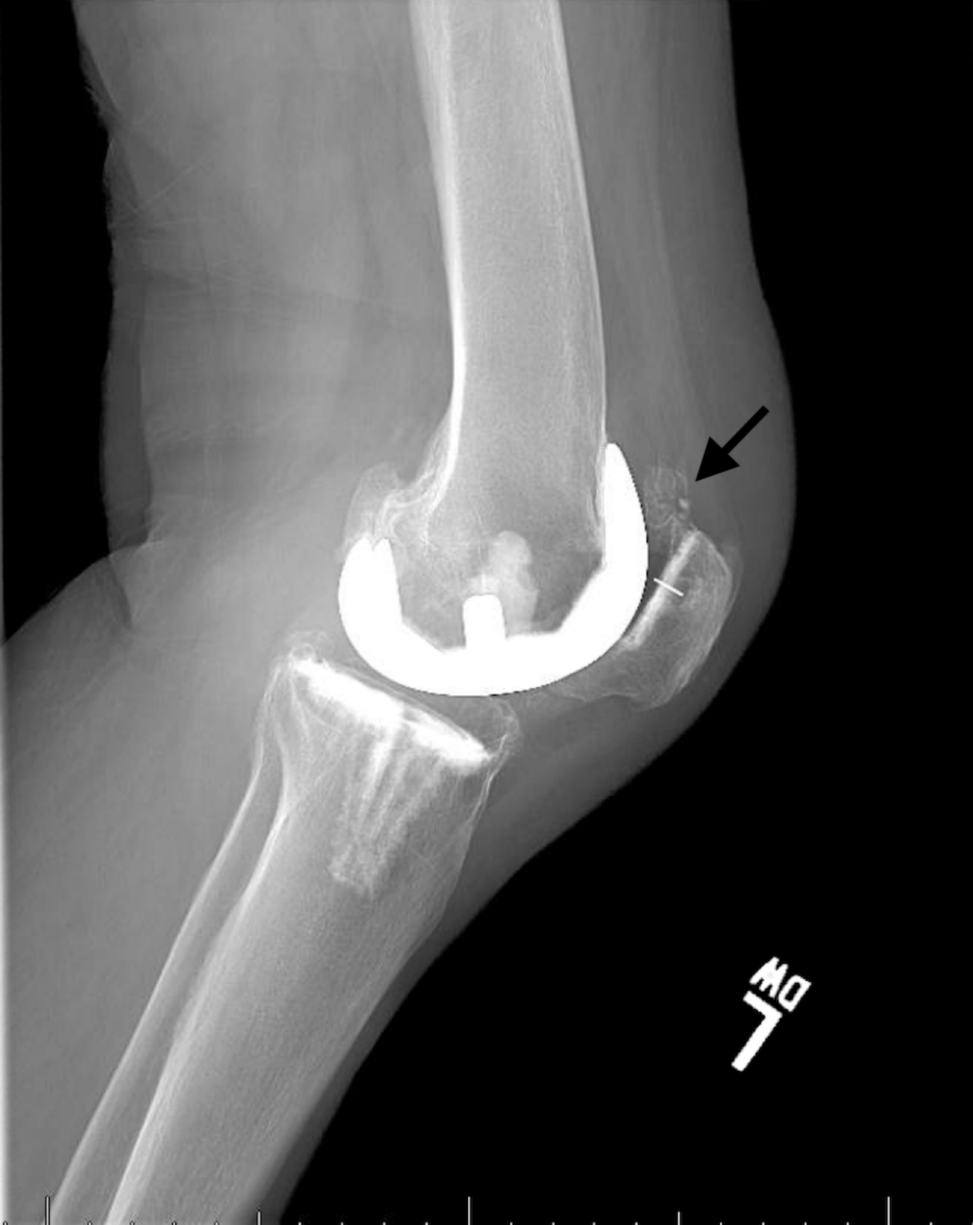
Lateral radiograph of left knee at presentation showing large osteochondral bodies in suprapatellar recess
